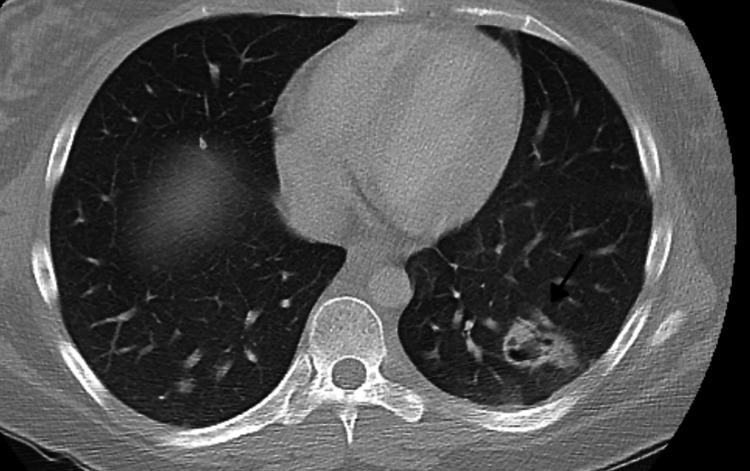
Computed tomography (CT) of the chest Black arrow showing the cavitary lesion measuring approximately 3 cm in diameter
